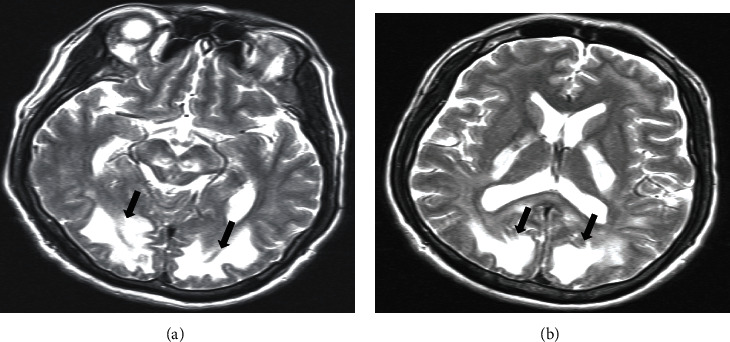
(a, b) T2-weighted Magnetic Resonance Imaging of the brain, showing abnormal signal changes in the periventricular white matter, severe in occipital lobe regions, with mass effect on pons and medulla.
